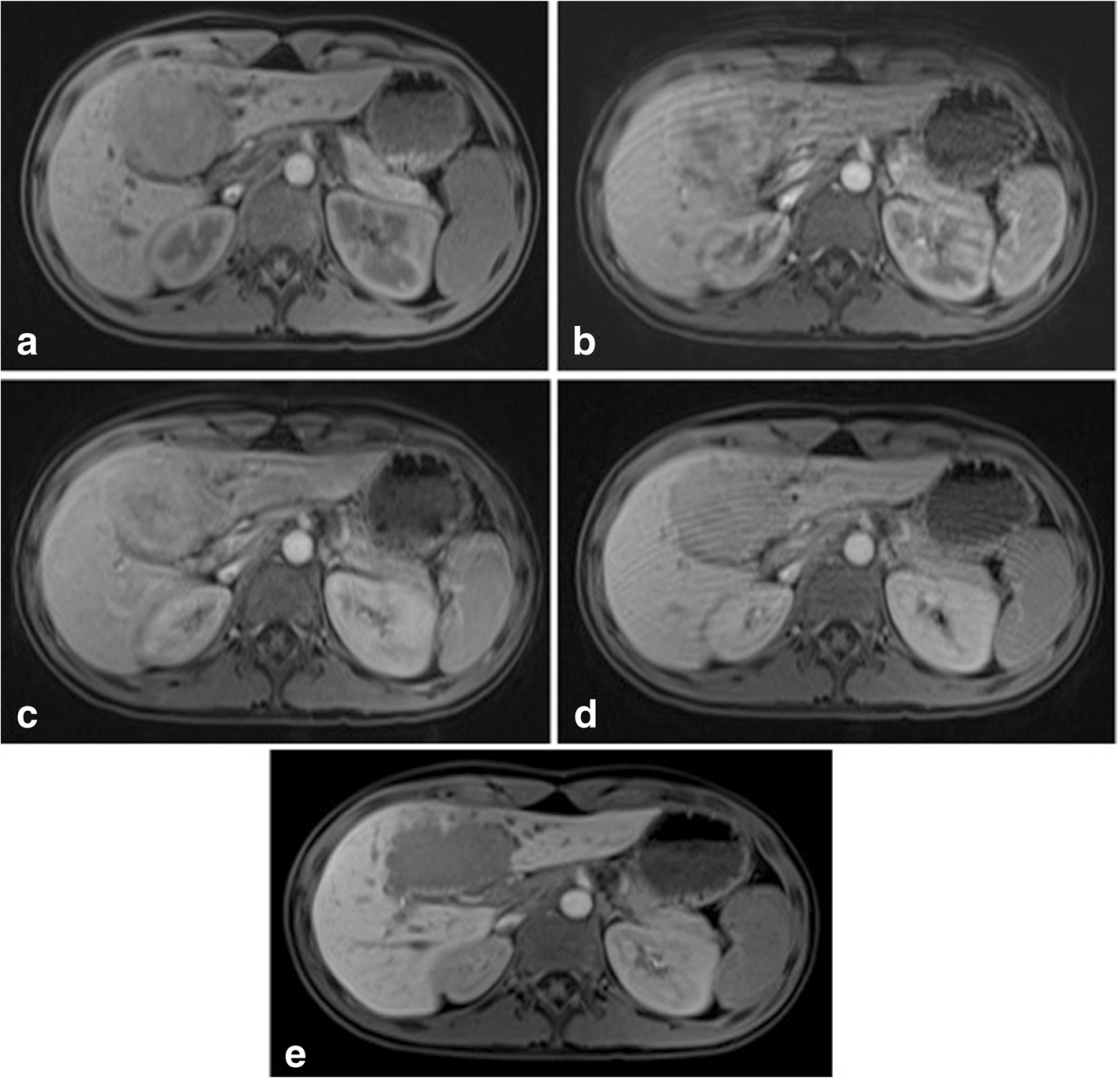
Fibrolamellar variant of hepatocellular carcinoma in a 15 year old patient. The hepatic lesion is hypointense to background liver parenchyma on (a) pre-contrast T1-weighted sequences with heterogenous internal enhancement after administration of gadoxetic acid in (b) arterial phase and (c) porto-venous phase imaging. There is gradual washout of contrast on the (d) 5 min delayed and (e) 20 min delayed imaging. Note the excretion of contrast material within the common bile duct allowing localisation of the mass and its relation to the biliary system, aiding surgical planning
